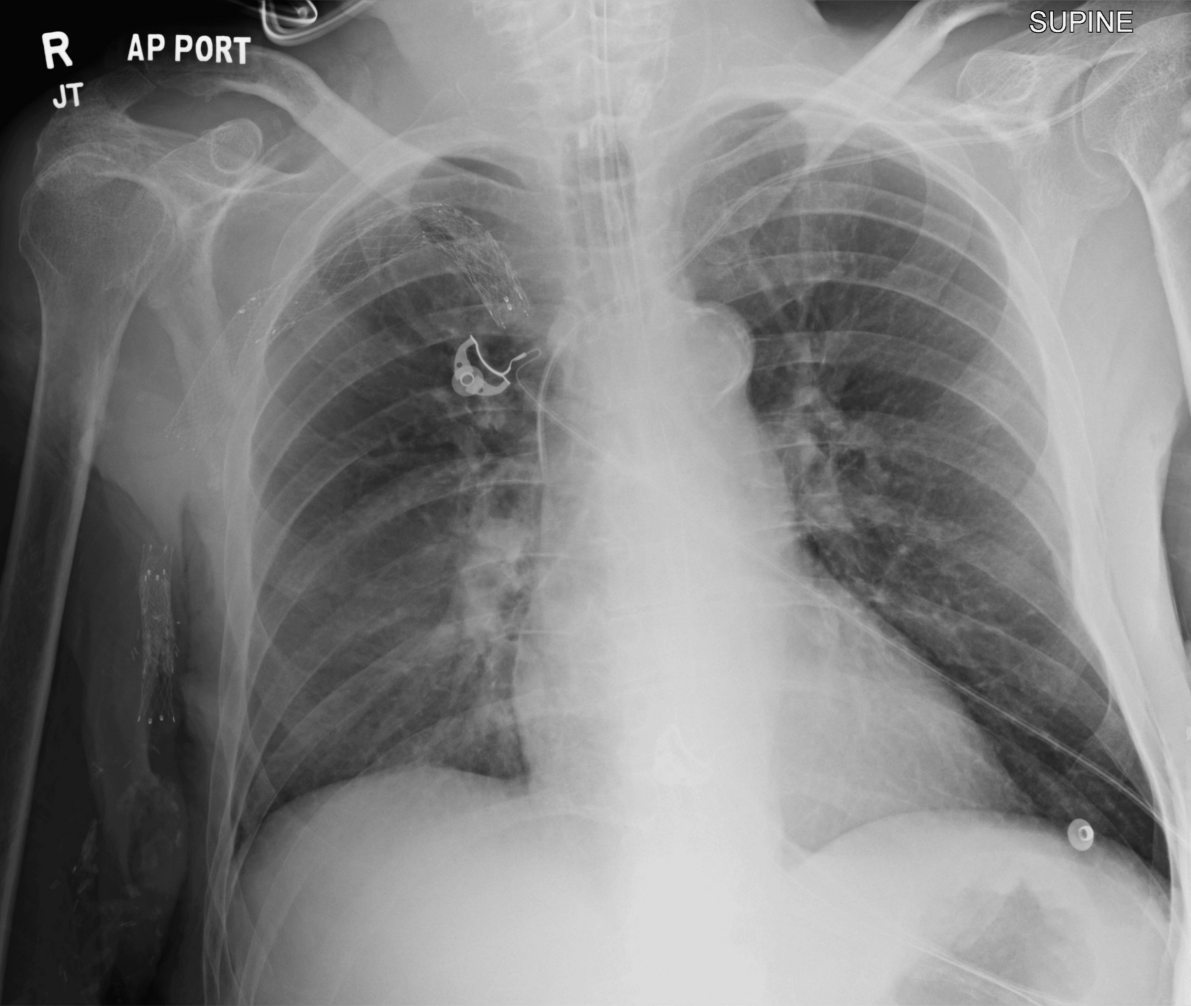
Chest X-ray showing clear lungs. The heart is normal in size. The endotracheal tube is seen with the tip 3.3 cm above the carina. The nasogastric tube is seen with the tip in the stomach.
